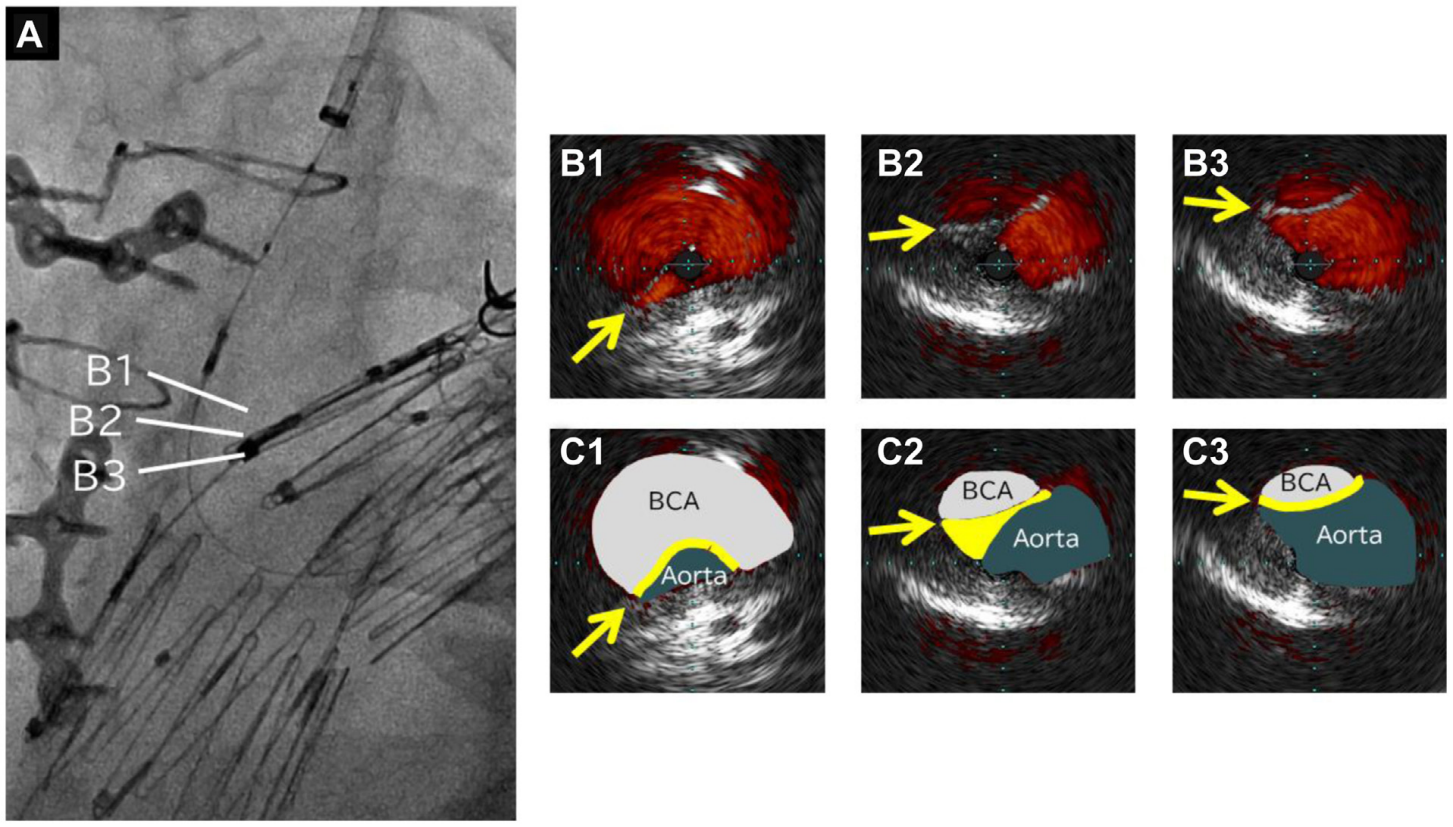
A, Angiography of the intravascular ultrasound catheter. B, Intravascular ultrasound images arranged in sequence from the brachiocephalic artery (BCA) toward the aorta: B1-B3 and C1-C3 show the cord-like structure stenosing the BCA (*arrow*).
